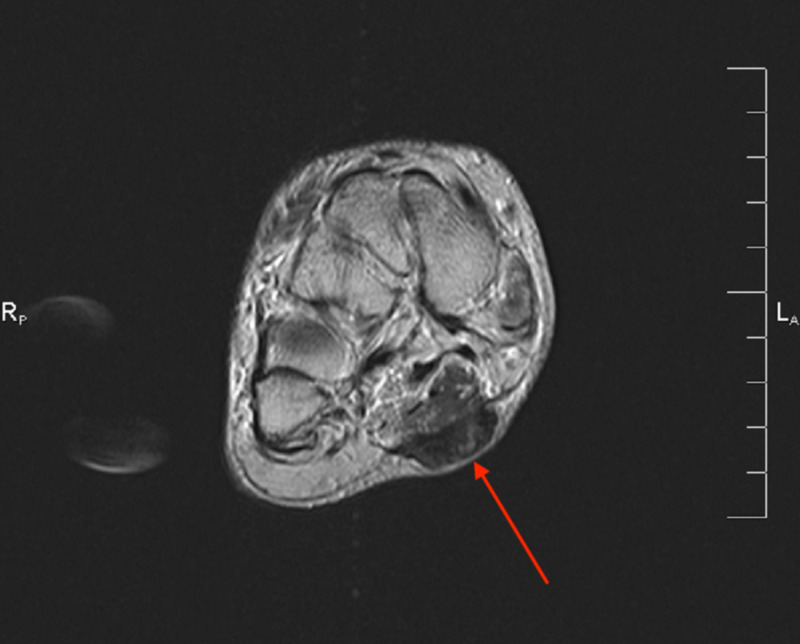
Case 3 MRI showing the axial T2 weighted image of the right foot demonstrating a large plantar fibroma extending from the central cord into the subcutaneous tissue of the plantar surface of the foot.
